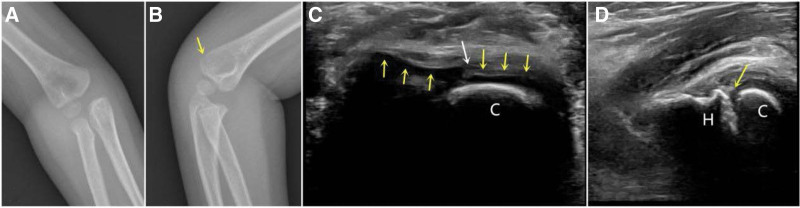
(A) A 4-yr-old boy, a slightly avulsion fracture of the lateral condylar of humerus can be seen on the anteroposterior film (arrow). (B) A slightly avulsion fracture was latent on the lateral film. (C) The transverse ultrasound showed the accumulated articular cartilage gap (white arrow) and step-off (yellow arrow) of the fracture. (D) The longitudinal ultrasound showed the fracture gap (yellow arrow) was much clearer on ultrasound than the ones in X-ray. C = capitellum, H = humer.
